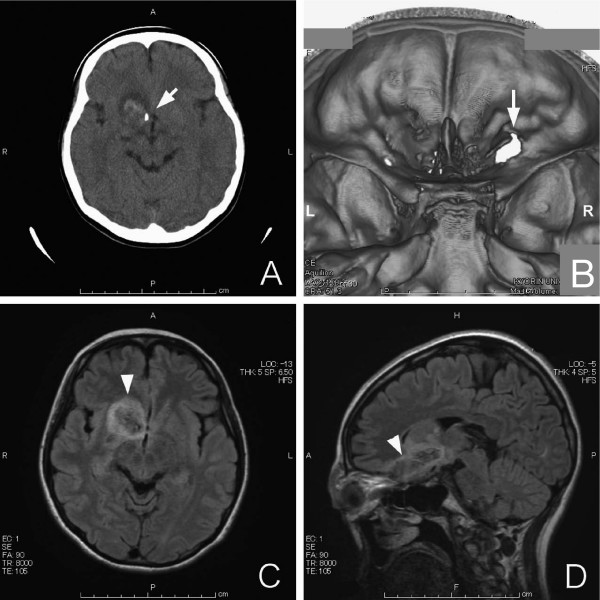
Computerized tomographic and magnetic resonance images of the patient's brain. A) This computed tomography (CT) scan shows incarcerated orbital bone fragment (arrow) and brain contusion. B) This three-dimensional CT scan shows a window defect caused by a fracture (arrow) of the superior orbital wall. C) This magnetic resonance image (MRI) shows the brain edema in greater detail (arrowhead). D) This MRI sagittal section shows where the umbrella tip had penetrated through the periorbital puncture (arrowhead).
